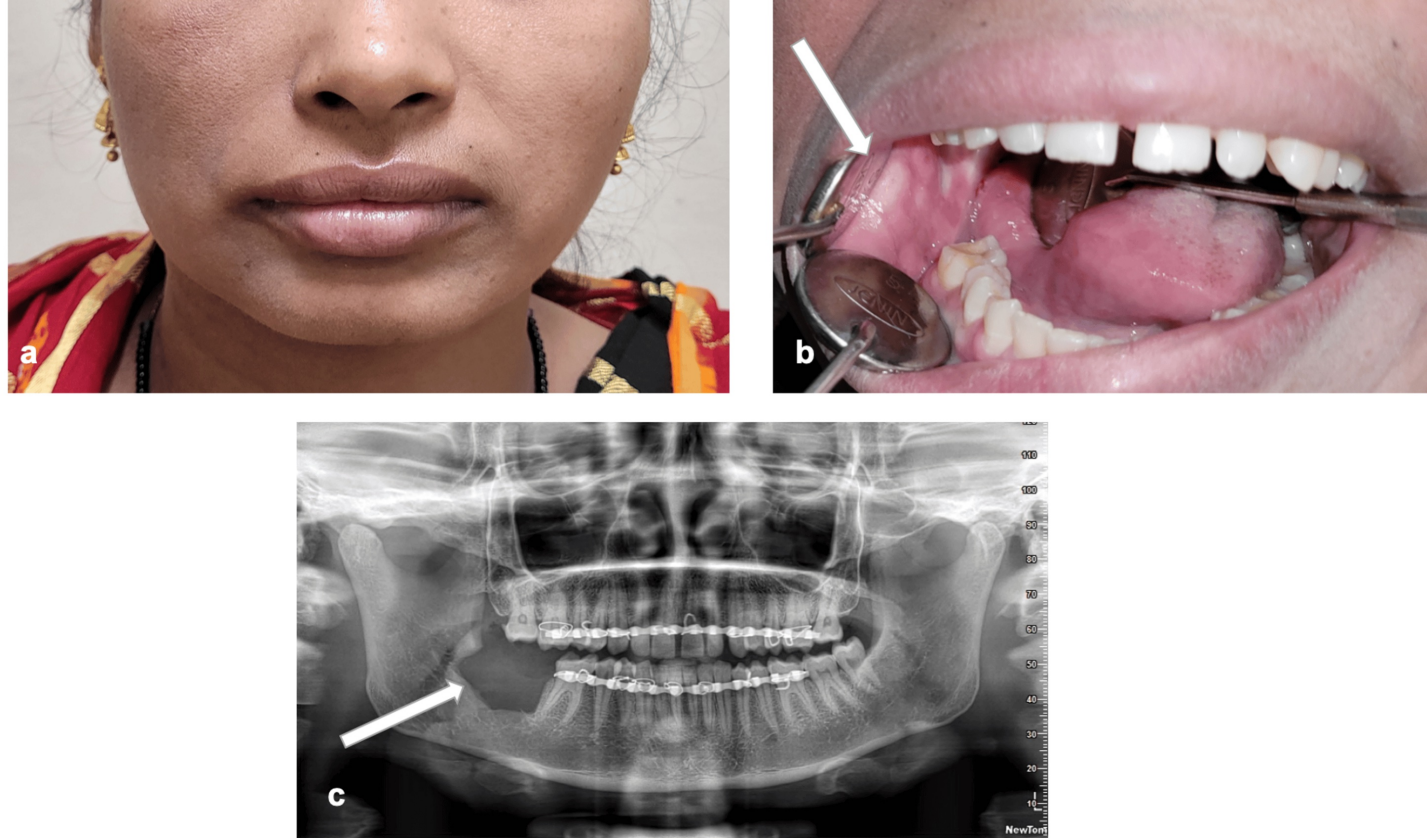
a and b: Extraoral and intraoperative postoperative images. c: Postoperative OPG OPG: Orthopantomogram
